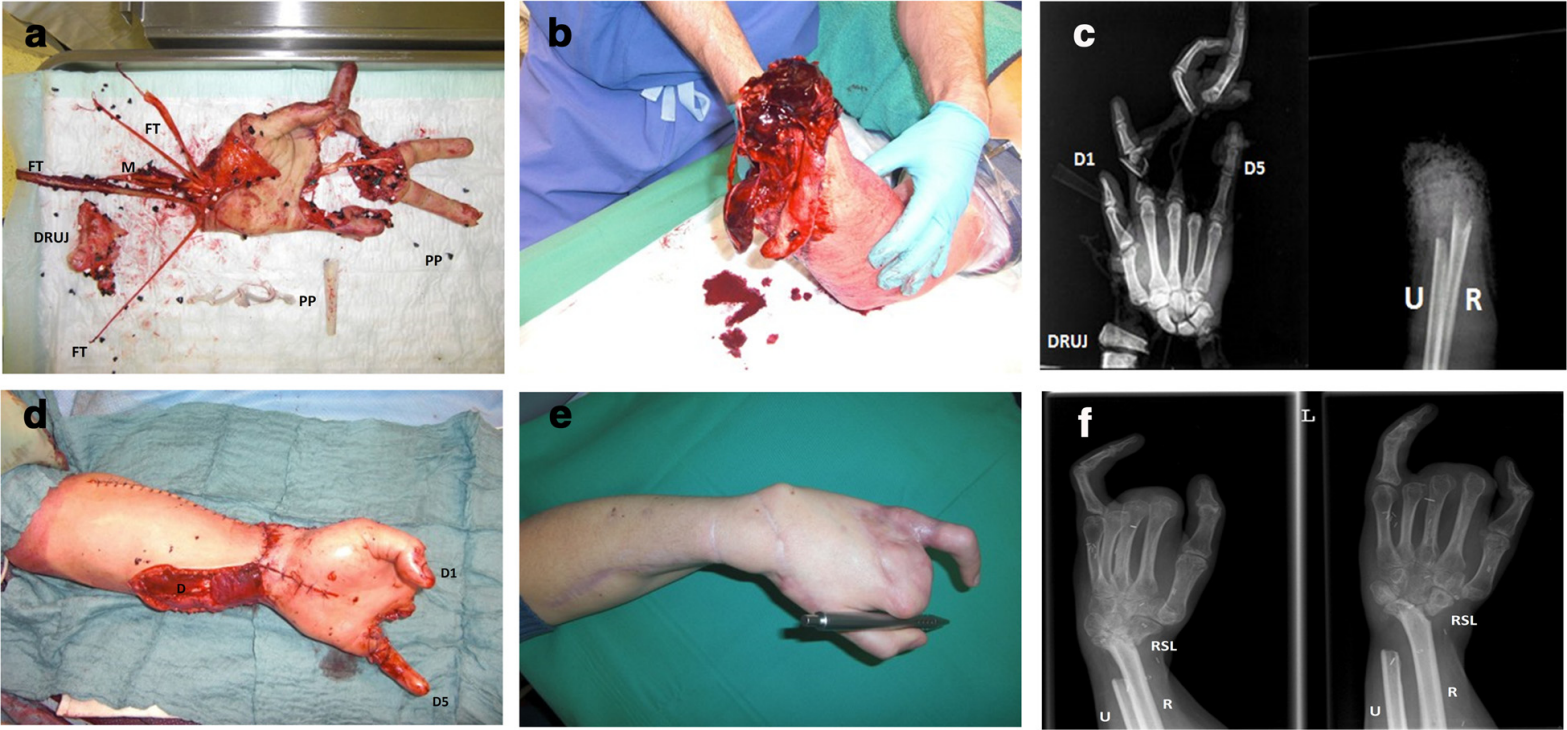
a: Amputated left hand of a 26 year old male at the level of the wrist preoperatively with a multilevel and avulsion injury through a plastic pellet machine. It shows additional subtotal amputation of the index finger and complete amputation of the middle- and ringfinger. The thumb was not injured; the little finger had a radial soft tissue defect. (PP) = black/white plastic pallets. (DRUJ) = distal radioular joint. (FT) = Flexor tendons. (M) = N. medianus. b: Preoperative situation of the stump of the distal forearm of the same 26 year old male. c: X-Rays of the left lower upper extremity and the left amputated amputated hand. (D1) = digit 1. (D5) = digit 5. The left picture shows a complete fracture of the distal radius and the distal ulna, with the distal radio ulnar joint lying transverse (DRUJ). The right picture shows the lower upper extremity with a fracture of the radius (R) and the ulna (U). d: Replanted hand postoperatively: thumb (D1) and little finger (D5) could be preserved, the index, middle and ringfinger were not re-plantable, the remaining defect (D) was temporarily covered by a negative pressure wound dressing and in a second operation 5 days later covered with split-skin-grafts. e: Replanted hand 9 months postoperatively: the patient is able to perform minimal flexion and adduction of the thumb in order to grip objects. f: X-Ray of the replanted hand 9 months postoperatively. (U) = ulna. (R) = radius. (RSL) = atypical RSL-arthrodesis of the left wrist
